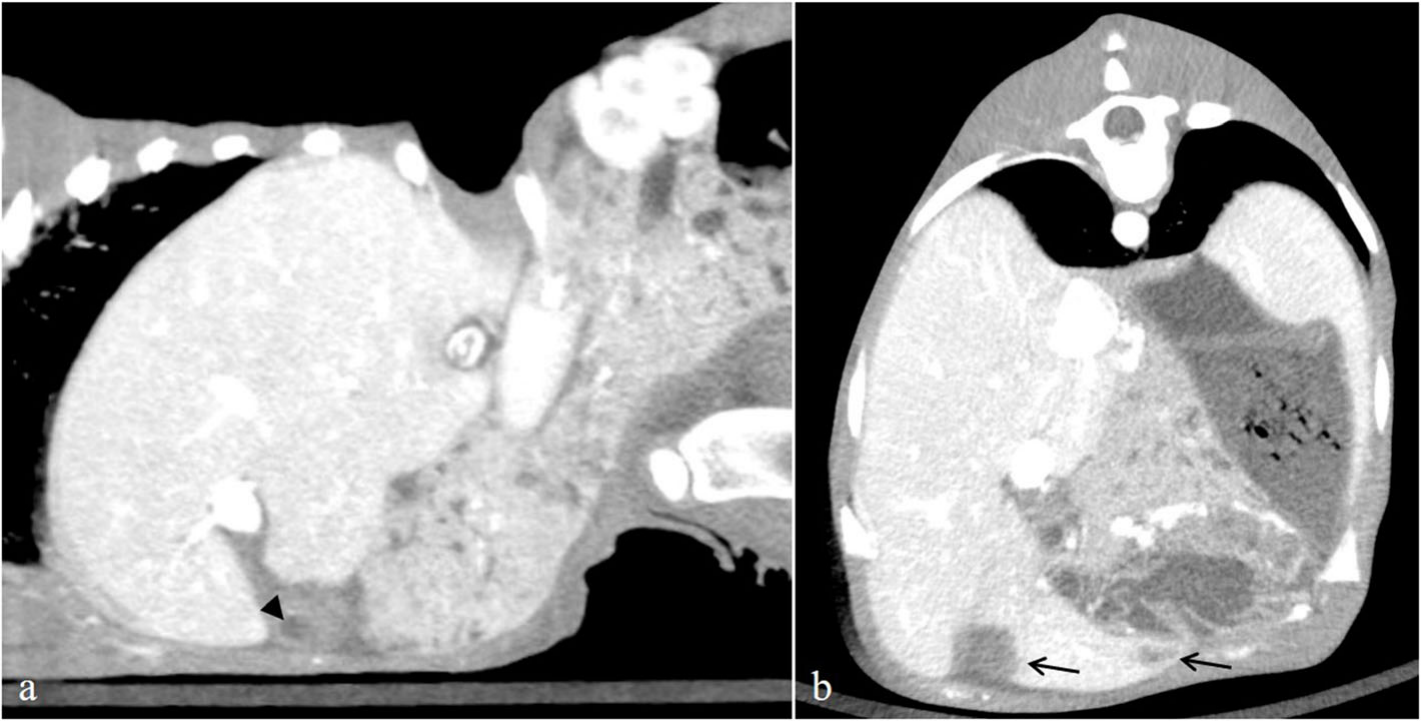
Contrast-enhanced CT images in the liver in the calf. a Sagittal image revealed an umbilical vein containing fluid (arrowhead). b Transverse image revealed multiple unenhanced lesions, most likely abscesses, which were confirmed in the liver (black arrow). CT, computed tomography
